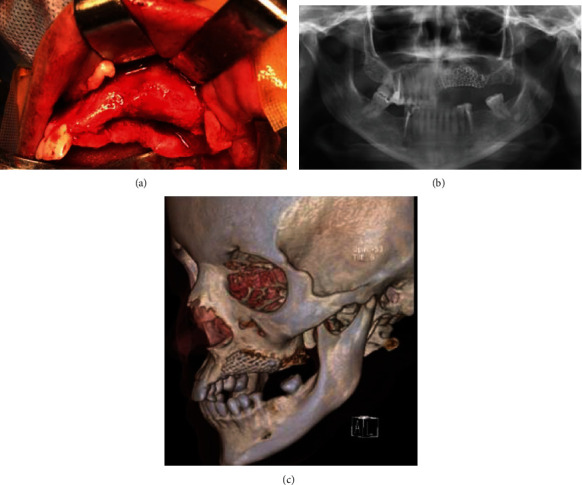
(a) Photographs showing the postoperative view of the maxillary defect. (b, c) Postoperative computed tomography and radiographic evaluation.
